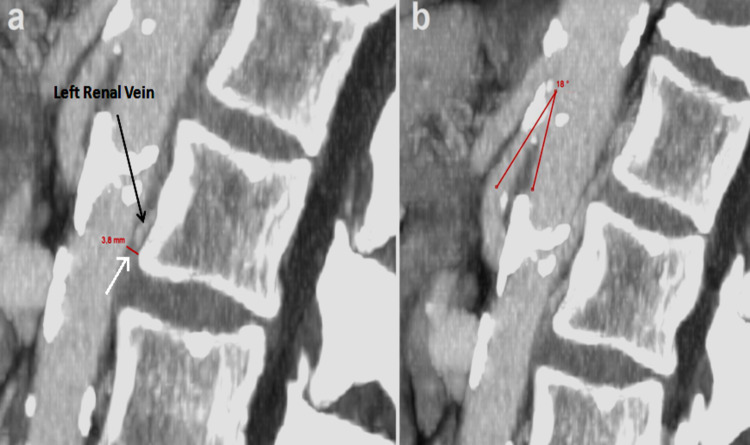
CT shows sagittal plane reconstructions (a) In sagittal view, it is possible to measure aorto-vertebral distance (arrow) which appears considerably reduced (3.8 mm). (b) In sagittal view, the aorto-mesenteric angle can be measured, which appears reduced (18 degrees), and superior mesenteric artery originating from aorta can be seen with an acute angle "beak sign."
